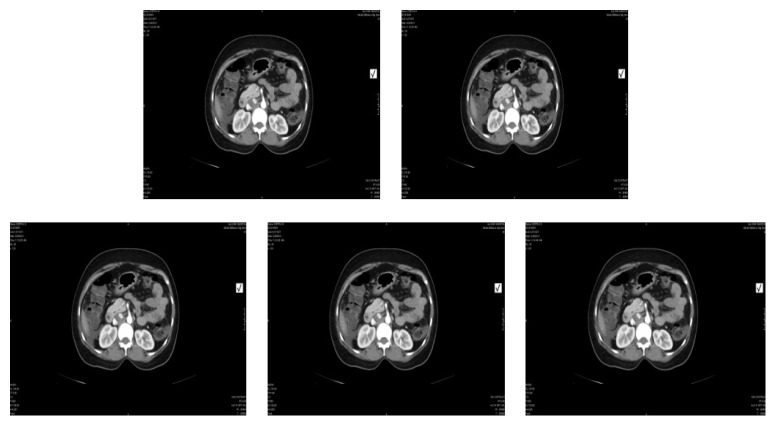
CT scan (abdomen and pelvis).
